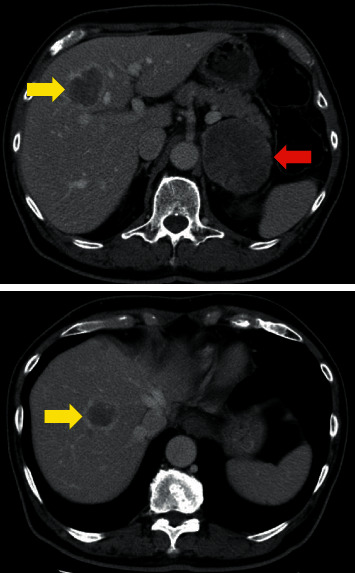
Abdominal computed tomography scan. The red arrow indicates the left adrenal tumor (diameter, 7 cm). The yellow arrows indicate multiple liver tumors (diameter, ∼4 cm).
